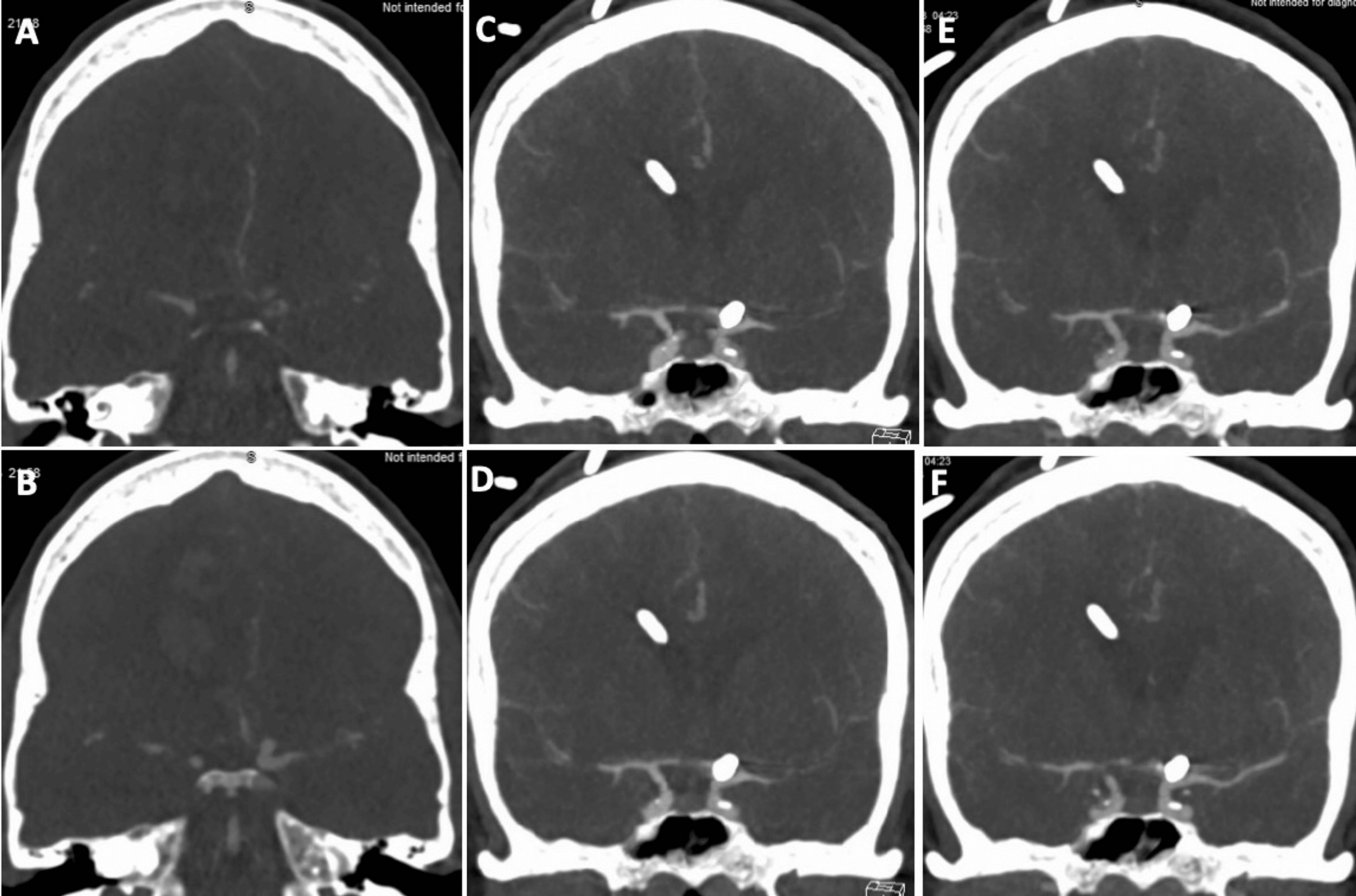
Initial CTA of the right (A) and left (B) anterior hemispheres, CTA demonstrating vasospasm in the right (C) and left (D) anterior hemispheres on day 6, and follow-up CTA demonstrating improvement in vasospasm in the right (E) and left (F) anterior hemispheres two days after initiating albumin and cilostazol therapy in patient 3 CTA: computed tomographic angiography
